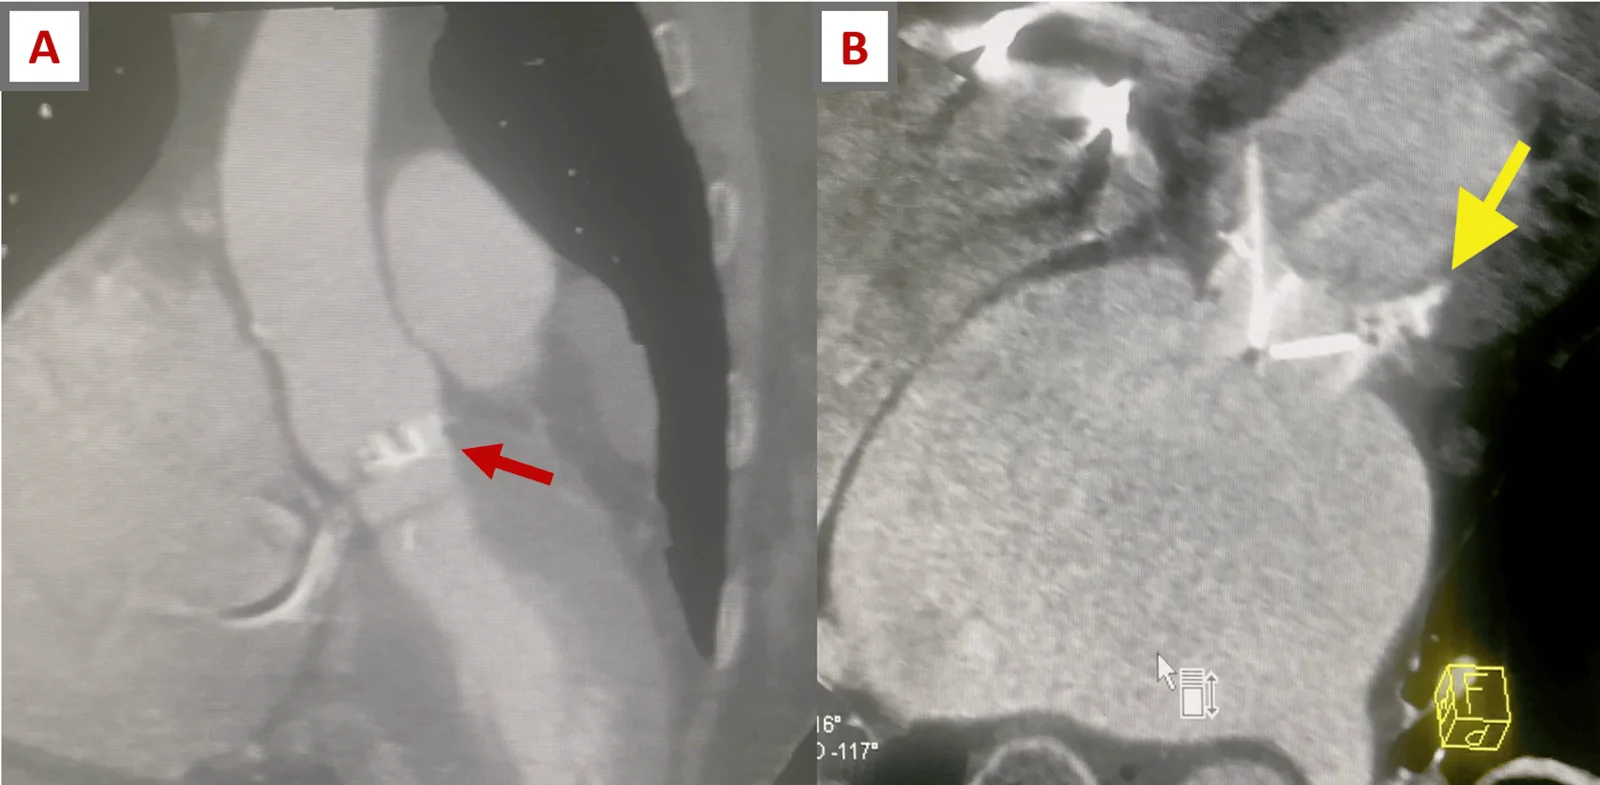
Cardiac CT of the mechanical valves in the mitral and aortic positions. (A) The red arrow indicates a dense subvalvular pannus located on the ventricular side of the mechanical aortic prosthesis. The lesion appears as a focal fibrous tissue overgrowth extending from the prosthetic ring toward the leaflet, resulting in restricted leaflet motion and prosthetic valve obstruction. (B) The yellow arrow indicates a dense pannus formation adjacent to the lateral aspect of the mitral prosthetic disc. The fibrous tissue extends toward the leaflet and contributes to impaired disc mobility and prosthetic valve obstruction.
